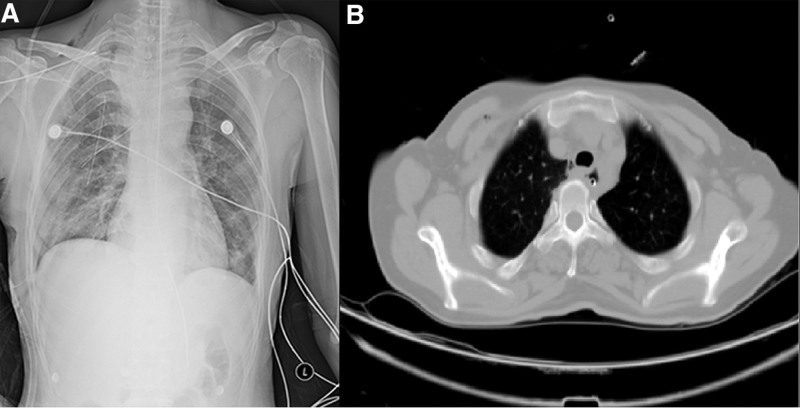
(A) On the first postoperative day, bedside orthographic film; (B) chest CT on day 5 after surgery.
